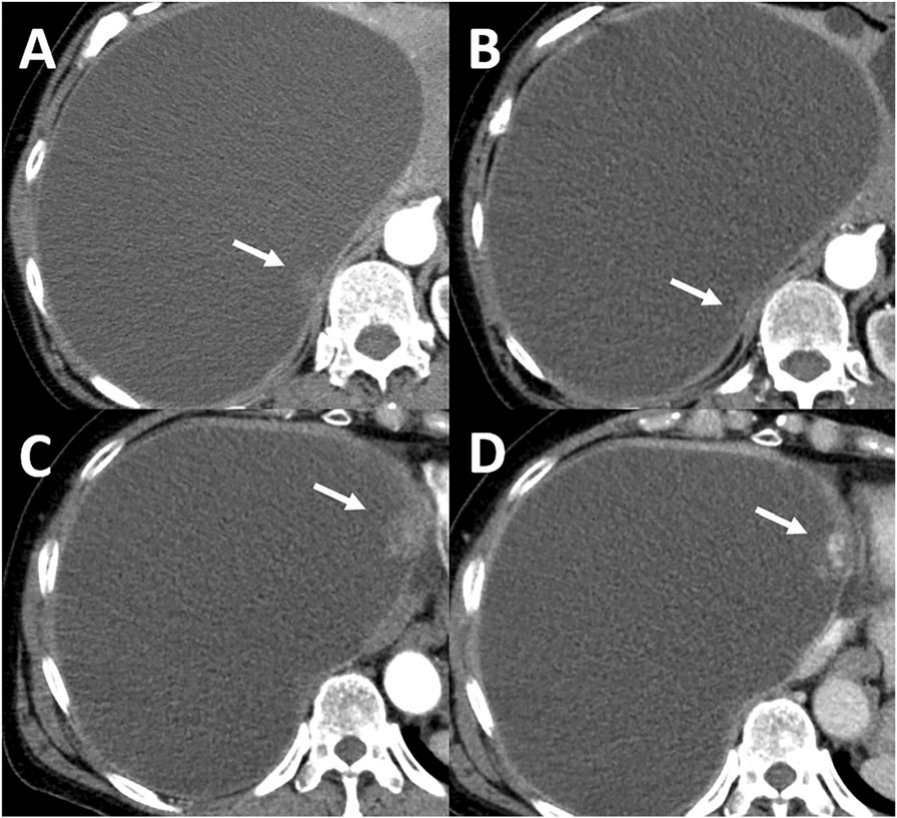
CT images of mural nodules in giant cyst. a Plain CT showed a dorsal unenhanced mural nodule within the cyst wall (arrow), b which did not change thereafter (arrow). c Enhanced CT showed a ventral mural nodule with weak enhancement in the early phase (arrow) and d with centripetal prolonged enhancement in delayed phase (arrow)
